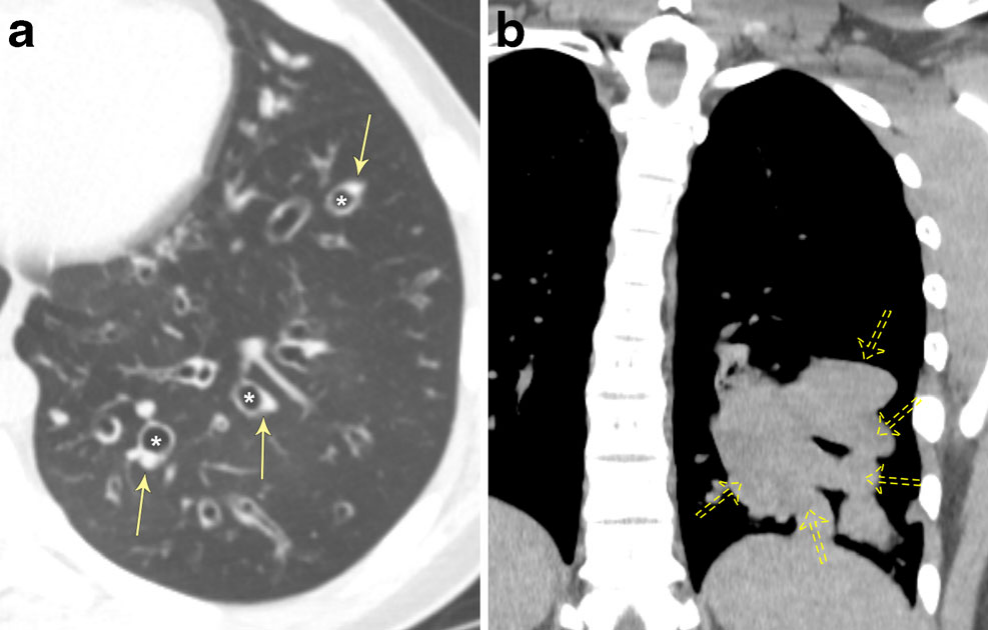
Classic imaging signs of bronchiectasis. a Signet ring sign is a CT finding referring to a markedly enlarged bronchus (*) mimicking a ring and its adjacent normal accompanying pulmonary artery representing the signet emblem of the ring (*arrow*). b Finger-in-glove sign describes the presence of mucous or fluid impaction within a dilated bronchus (*dashed arrow*) appearing as a radiopaque “finger” in the “glove” of the bronchus
